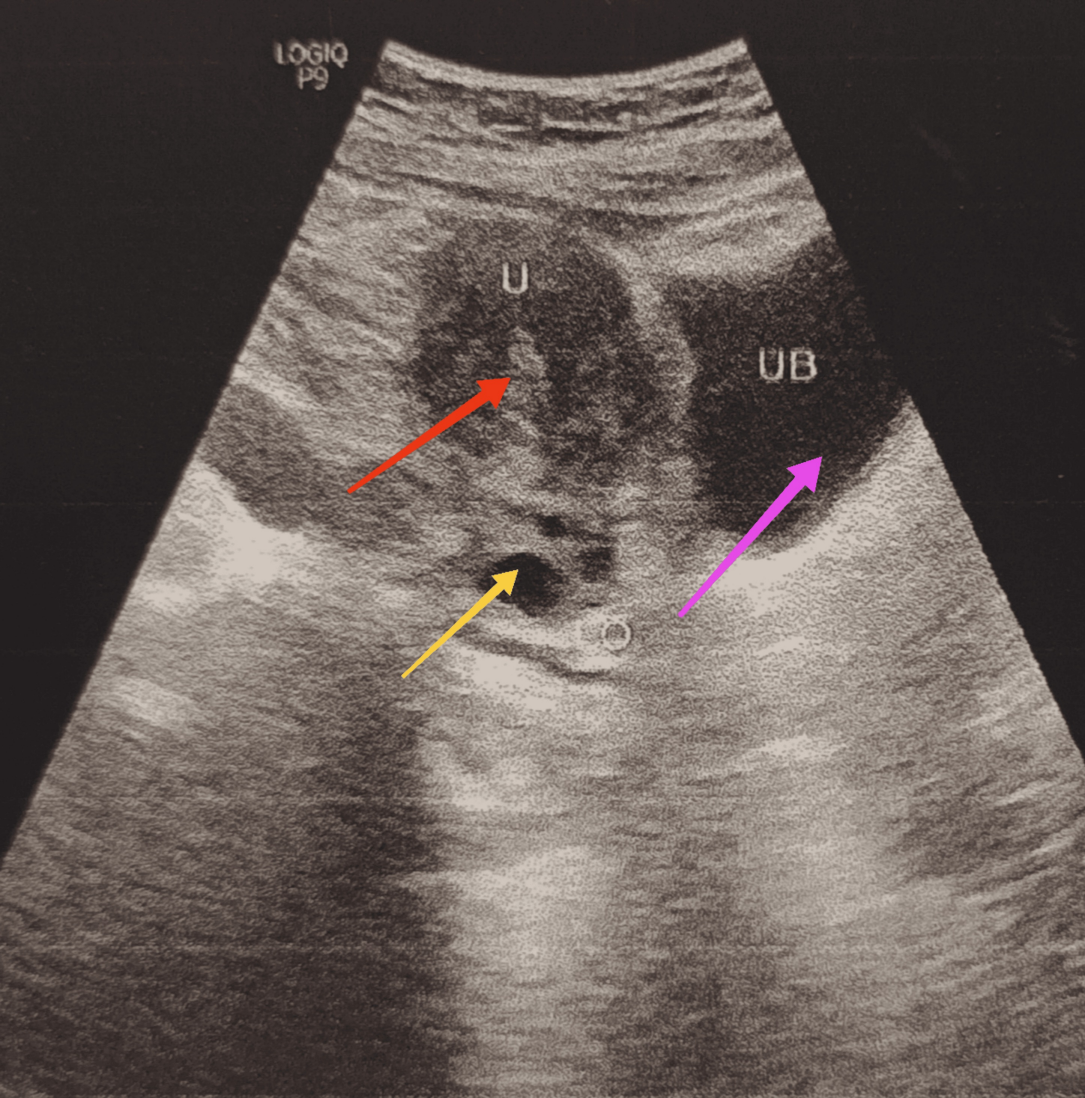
Sonography showing uterus and ovary. The red arrow shows the endometrium; the yellow arrow shows the left ovary; and the purple arrow shows the urinary bladder.
